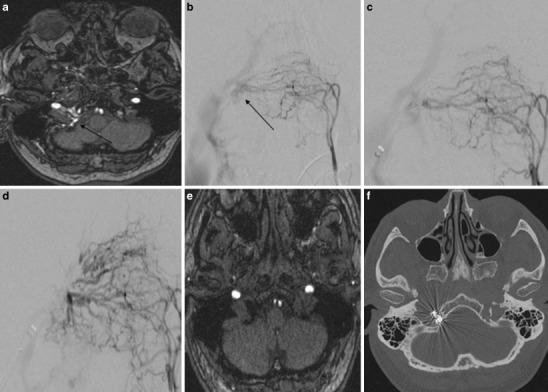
Case #3: a MR angiography source image from three-dimensional time-of-flight shows abnormal vascularization in the right hypoglossal canal compatible with a DAVF of the anterior condylar vein. b Anteroposterior (AP) view after injection of the left ascending pharyngeal artery shows a DAVF of the anterior condylar vein with a venous pouch located medially to the jugular bulb. c AP view after injection of the left ascending pharyngeal artery shows the microcatheter in the venous pouch and partial coil occlusion of the DAVF due to a transvenous approach. d AP view shows the complete occlusion of the DAVF; e MR angiography source image from three-dimensional time-of-flight shows no abnormal vascularization in the right hypoglossal canal anymore. f Axial computed tomography (CT) scan of the skull base shows the location of the coils in the right hypoglossal canal
